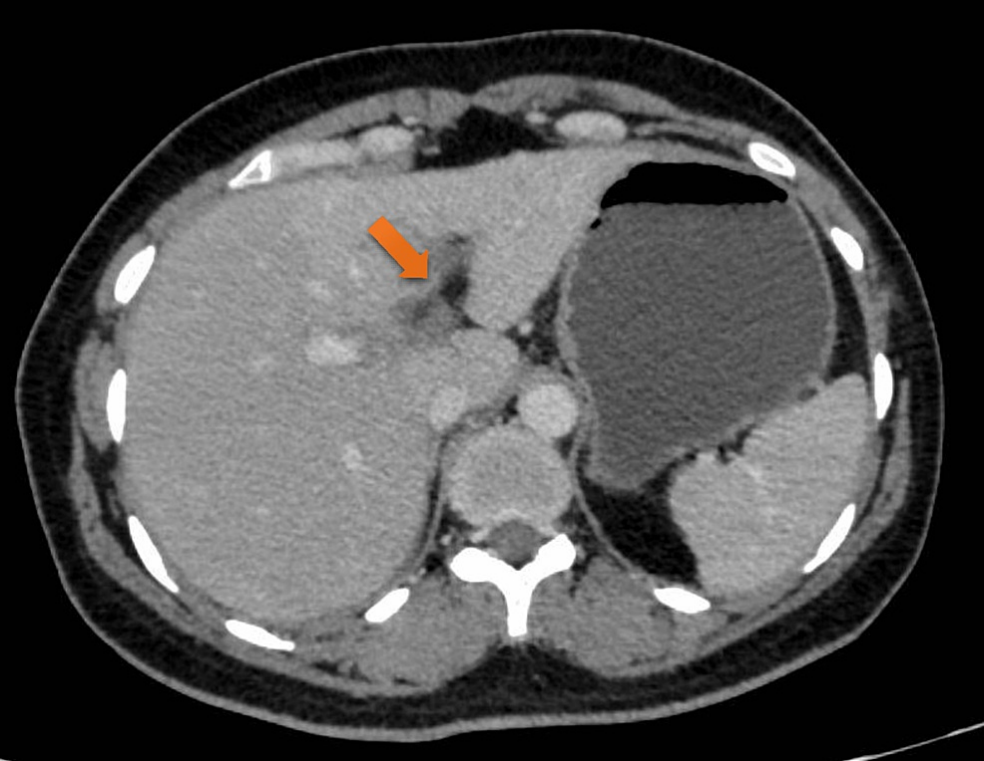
Abdominal CT scan showing a filing defect, indicating blockage of the left branch of the portal vein.
